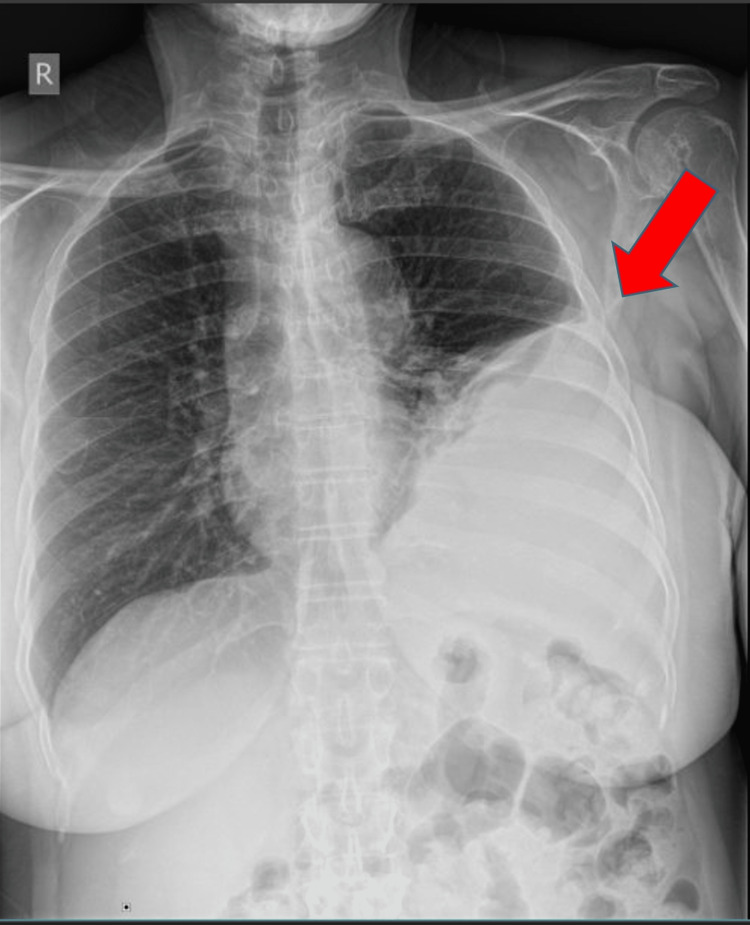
Chest X-ray showing an incidental pleural disease.
